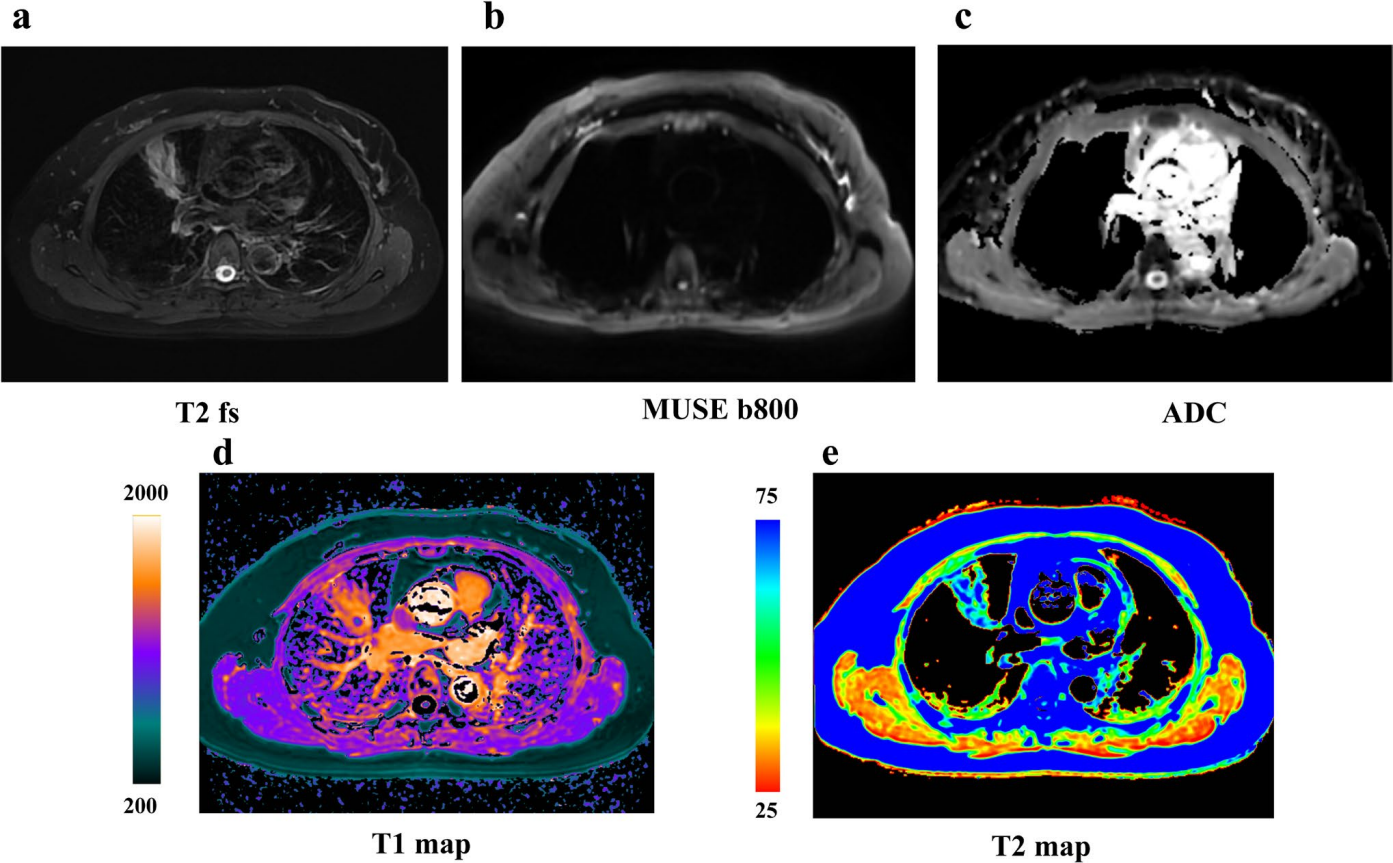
Female, 48 years old, solid nodule in the upper lobe of the right lung, approximately 20 mm in size, pathologically confirmed as an inflammatory lesion. (a) T2WI; (b) MUSE DWI (*b* = 800 s/mm^2^); (c) MUSE ADC; (d) T1 mapping (ms); and (e) T2 mapping (ms).
